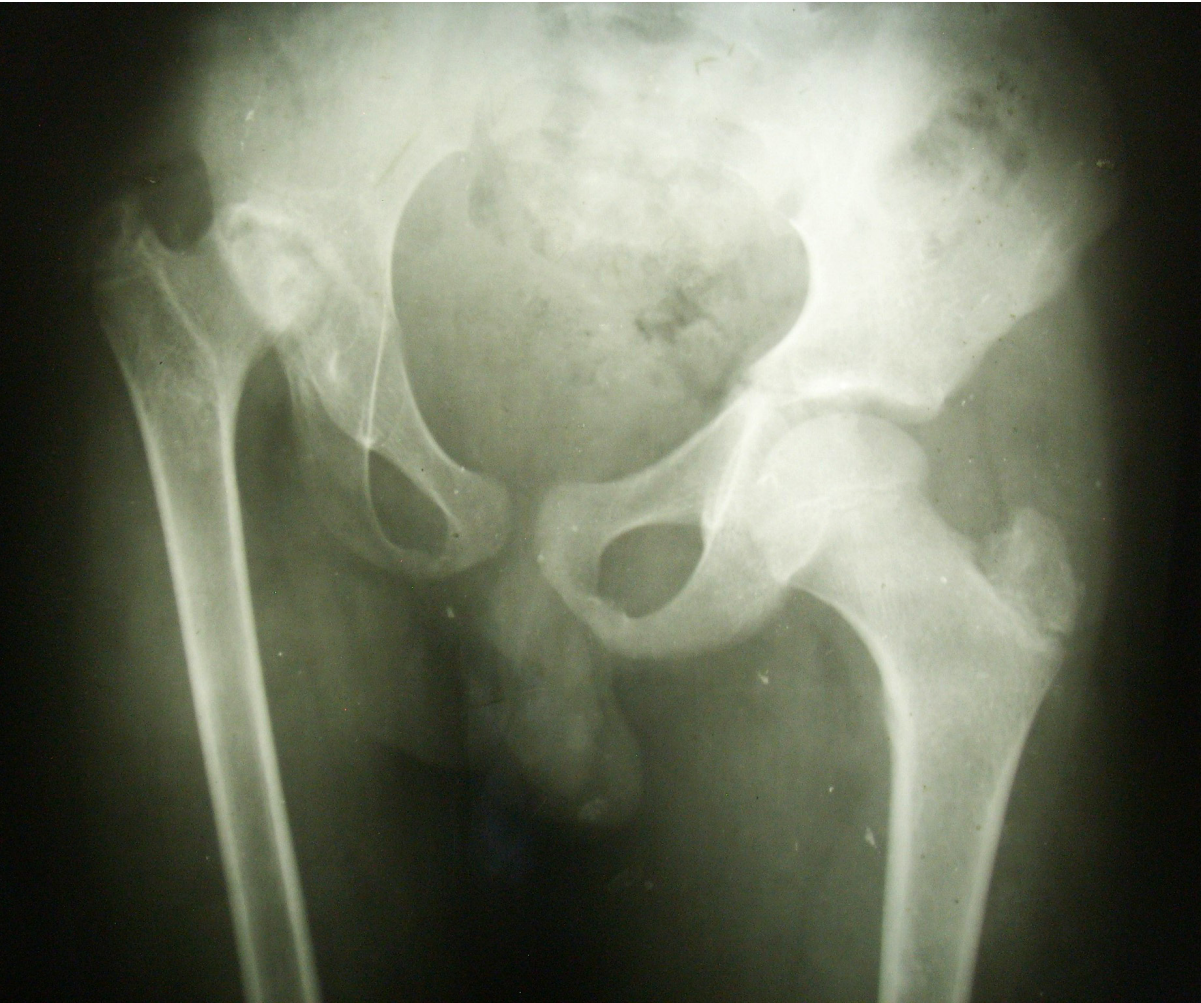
Radiographie. Le tiers supérieur de la tête fémorale est détruit, mais l’épiphyse reste relativement sphérique. Le cotyle est évasé; la tête fémorale est ascensionnée; le cintre cervico-obturateur est nettement perturbé mais le cintre cervico-iliaque est conservé, témoignant de l'absence d'excentration du fémur. Radiograph. The upper third of the femoral head is destroyed, but the epiphysis is still more or less spherical. The acetabulum is widened; the femoral head is displaced upward; there is no subluxation of the femoral head.
